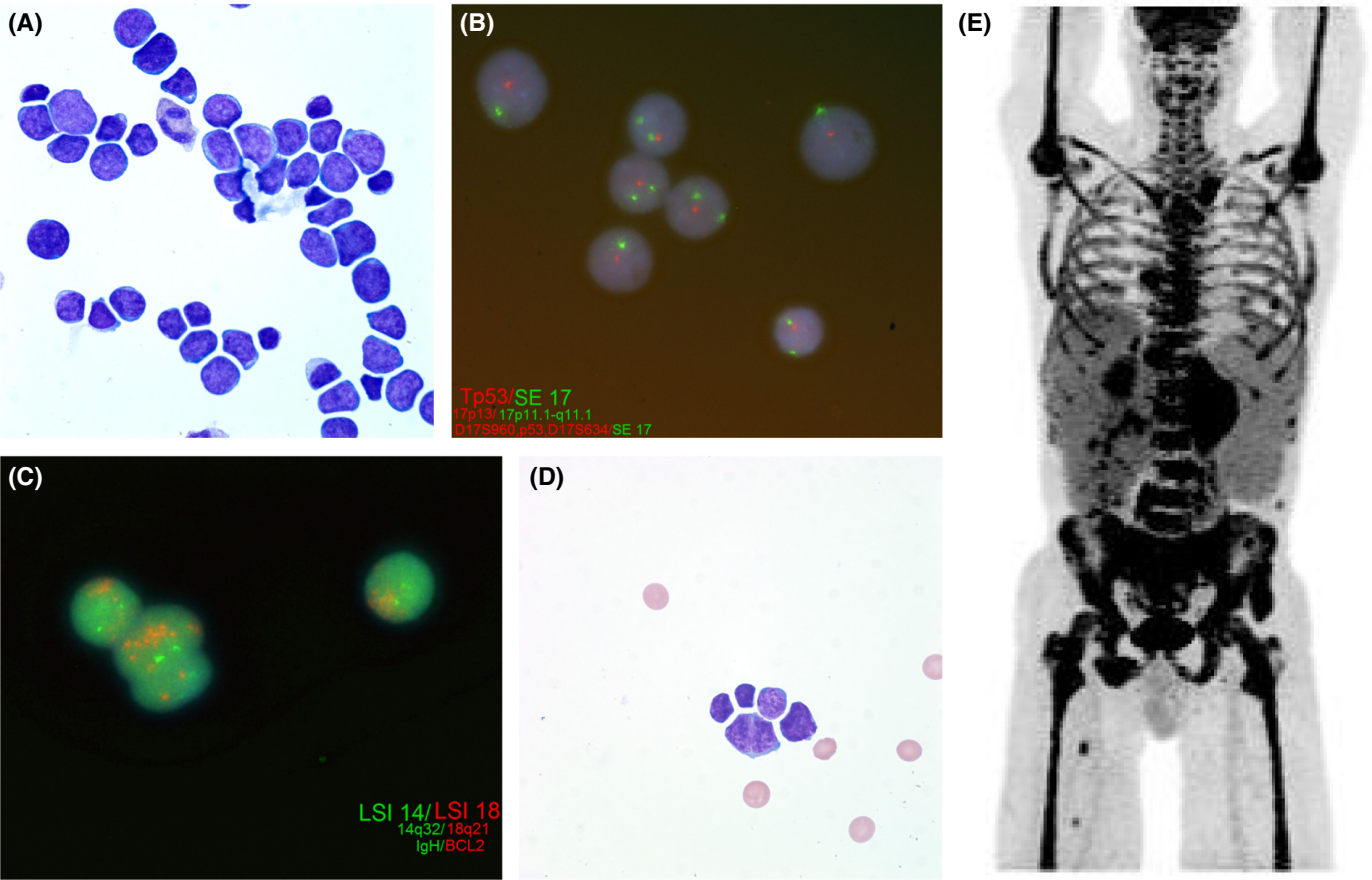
(A) Peripheral blood smear demonstrating leukemic involvement with DLBCL (B) FISH study demonstrating *TP53*/17p deletion in 83.5‐90% of involved cells (C) BCL2 amplification (red signals) (D) CSF involvement with DLBCL (E) PET/CT demonstrating widespread marrow and extranodal involvement.
